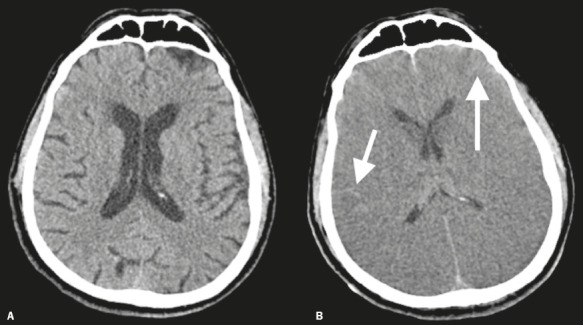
Brain CT of a 75-year-old man with COVID-19 and severe acute respiratory syndrome. A:
